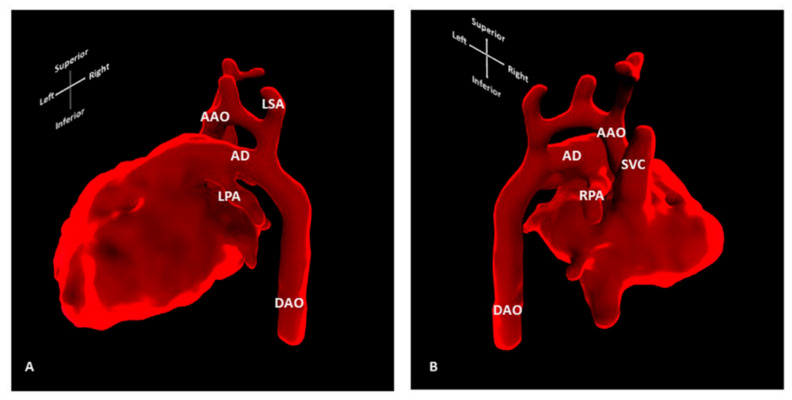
Three-dimensional foetal cardiac MRI showing high suspicion of CoA. Segmentation of motion-corrected 3D foetal cardiac MRI data, showing (A) left lateral and (B) right lateral view of the foetal heart. Foetal cardiac MRI was conducted at 30 + 2 weeks’ gestation for suspected coarctation of the aorta. Neonatal coarctation requiring surgical repair was confirmed after birth. AAO: Ascending aorta; AD: Arterial duct; DAO: Descending aorta; LPA: Left pulmonary artery; LSA: Left subclavian artery; RPA: Right pulmonary artery; SVC: Superior vena cava.
